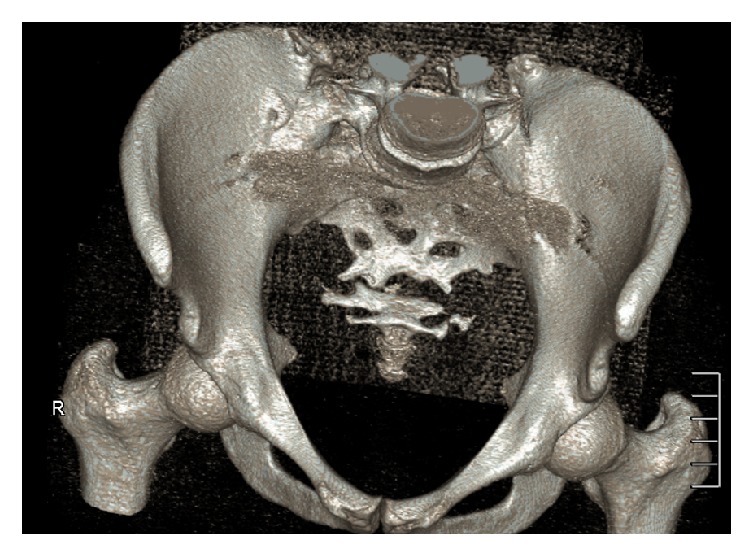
CT reconstruction of the pelvis showing the “neosacrum.”
